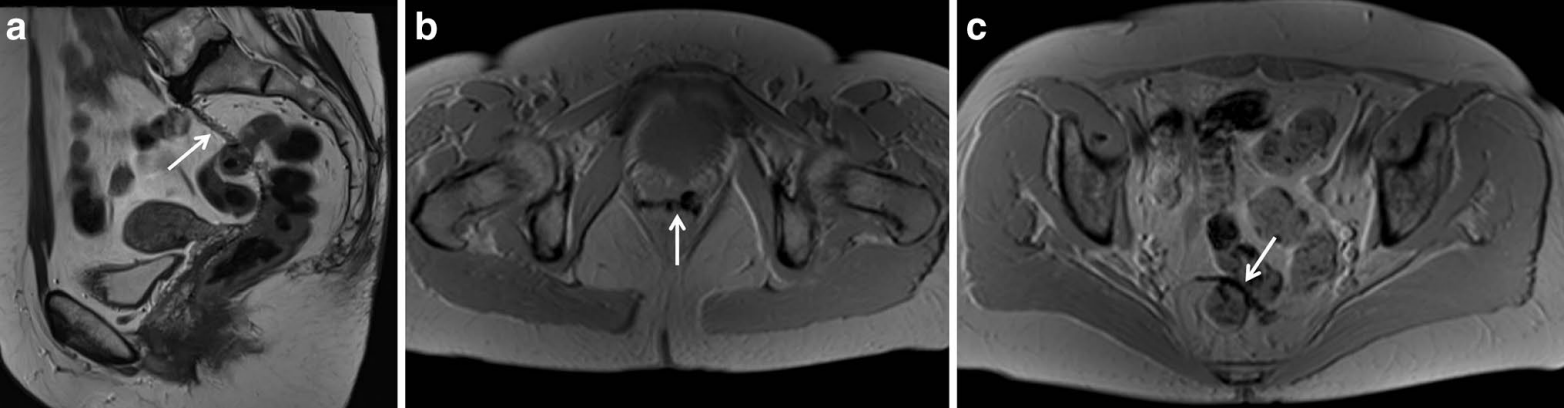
a The mesh is visualized in its full length on T2-weighted sagittal image. On T1-weighted flash images, the mesh is also well visualized close to the lower insertion point (b) and at the higher insertion on the rectal wall (c)
